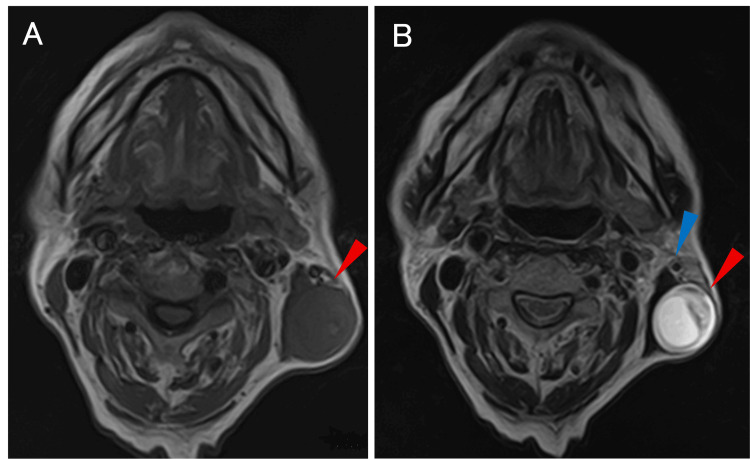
MRI findings (A) Axial T1-weighted image showing a mass with an isointense signal relative to muscle (red arrow). (B) Axial T2-weighted image showing a mass with high signal intensity and mild internal heterogeneity (red arrow), adjacent to the external jugular vein (blue arrow). MRI: magnetic resonance imaging
